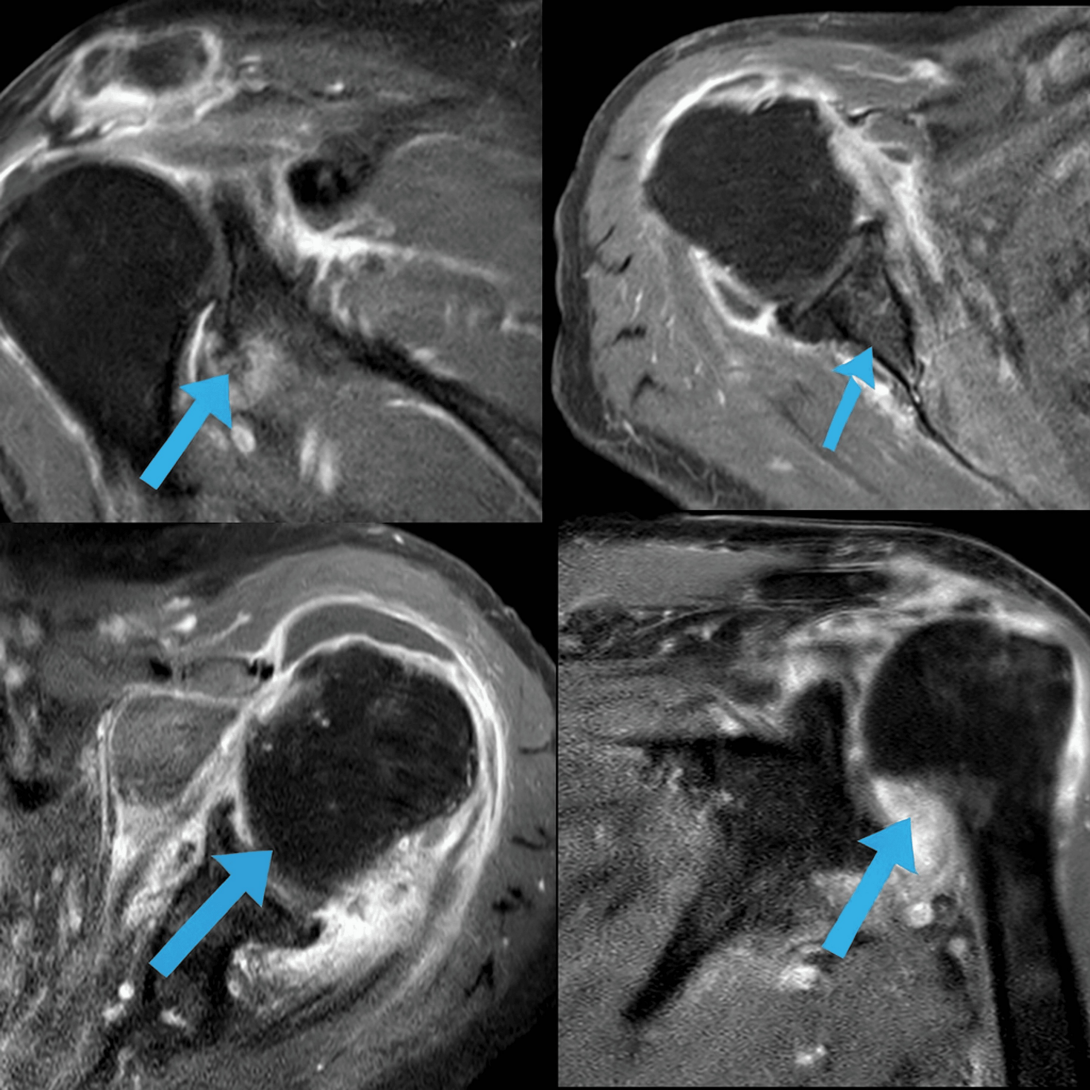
MRI of both shoulders Axial and coronal short tau inversion recovery (STIR) images display bilateral synovitis, bursitis, capsulitis, and peritendinitis characteristic of PMR, with no evidence of focal destructive lesions or infective processes.
